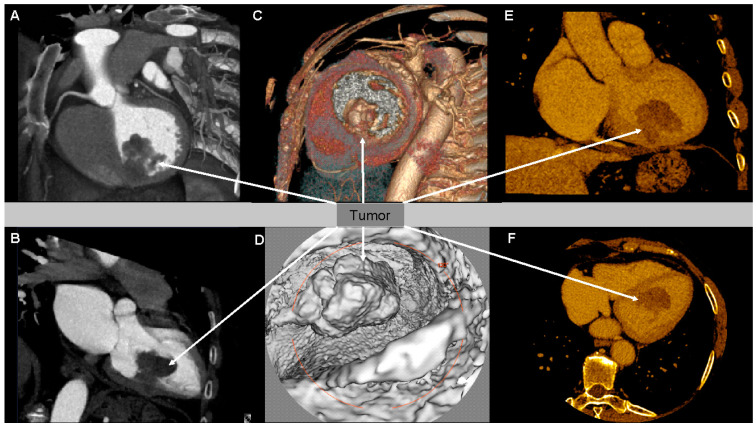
Multi-Slice Computed Tomography (MSCT) showing a pathological tumor mass connected with the posterior wall of left ventricle (LV) (arrows). (A) A 3D black-and-white reconstruction with the cutoff plane through the LV long axis; (B) a 3D black-and-white reconstruction with the cutoff plane through the LV short axis; (C) a 3D volume rendering reconstruction with the cutoff plane through the LV short axis; (D) virtual ventriculography of the LV in the navigator option axis; (E) a coronal multiplanar reconstruction with gold color scale; (F) an axial multiplanar reconstruction with gold color scale.
